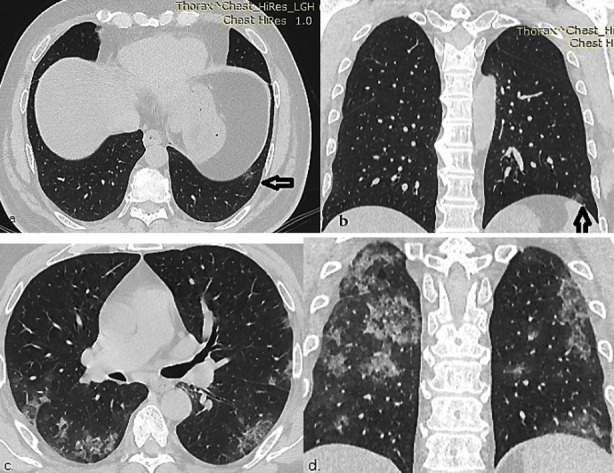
a- b) HRCT chest, axial and coronal images at 2^nd^ day of symptom shows unilateral focal GGO in left lateral basal segment. c-d) Axial and coronal of same patients on follow-up after 6 days shows bilateral GGO with surrounding rim of consolidation giving reverse halo sign in peripheral posterior distribution.
